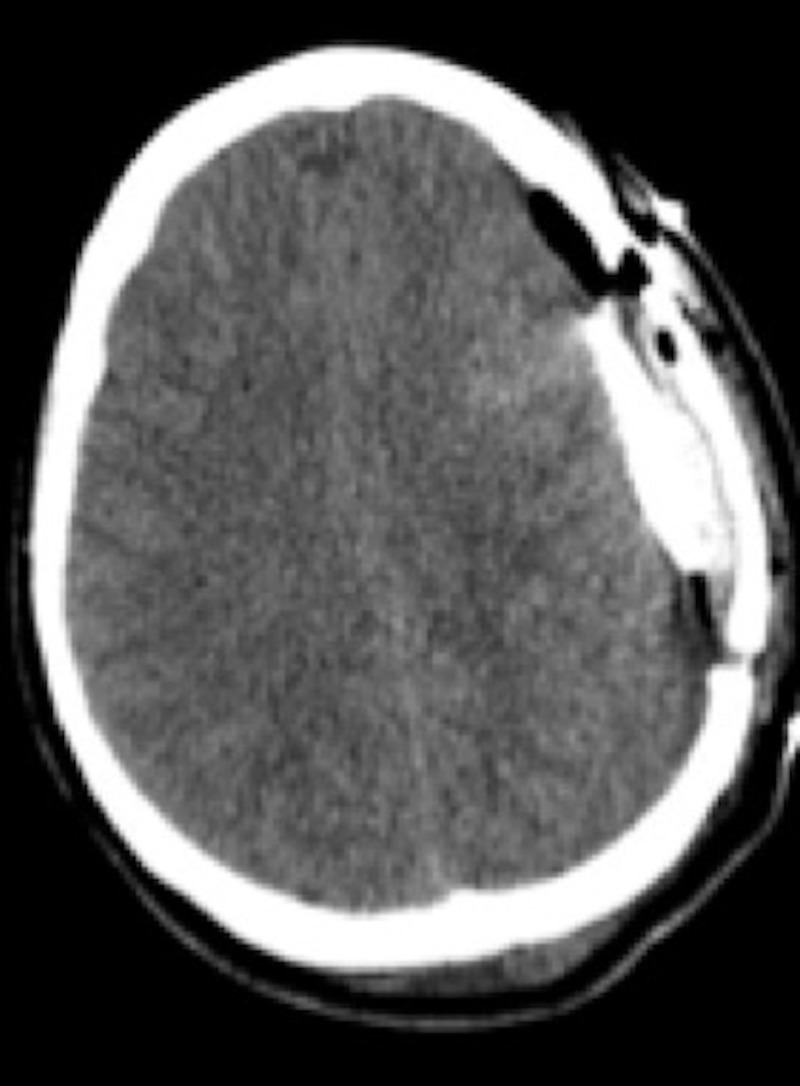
Postoperative noncontrast head computed tomography Axial view demonstrating the evacuation of a subdural hematoma and an arachnoid cyst with intra-cystic hemorrhage. A subdural drain (arrow) was placed intraoperatively to drain the residual subdural hematoma.
